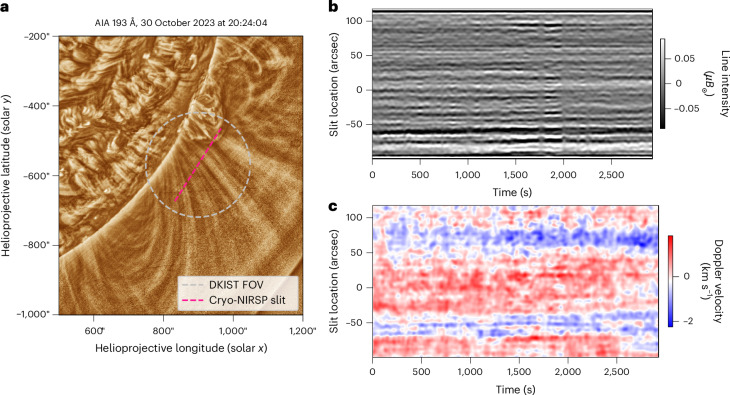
Overview of observations taken on 30 October 2023. a, Image of the solar corona taken with the Atmospheric Imaging Assembly onboard the Solar Dynamics Observatory in the extreme ultraviolet (193 Å channel) to provide context for the Cryo-NIRSP data. The DKIST field of view is indicated by the circle, and the approximate location of the Cryo-NIRSP slit is indicated by the red dashed line. b, Butterworth-filtered and unsharp masked line intensity data. The data reveal the fine-scale coronal structures that are subject to transverse displacements. Note that variations in seeing quality are visible as vertical bands. c, Doppler velocities from the sit-and-stare data (with the temporal mean subtracted from each time series). AIA, Atmospheric Imaging Assembly; FOV, field of view.
